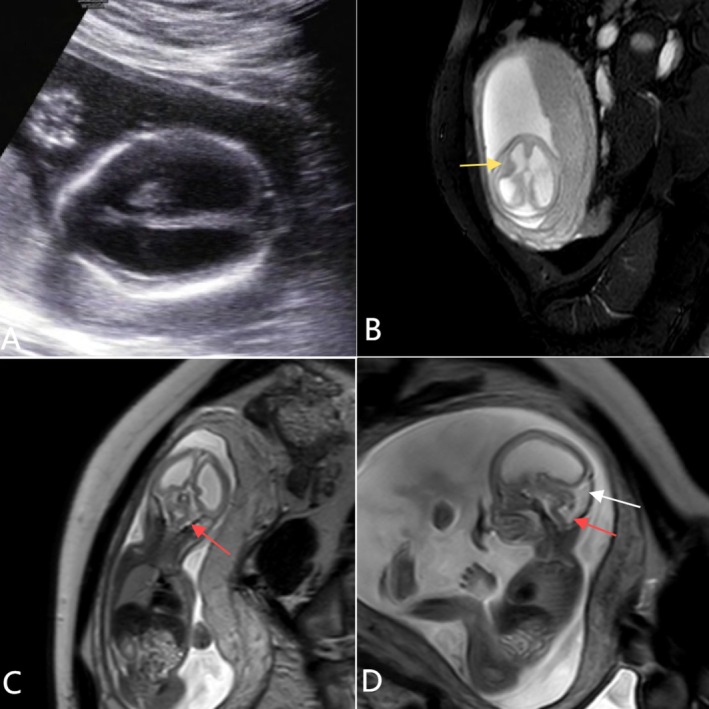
Images from Case 1. (A) US imaging at 19 weeks and 1 day shows severe triventricular hydrocephalus. Supratentorial sonographic images display an outer agyric echogenic band on the brain surface, along with a complete absence of the pericerebral space. (B–D) In vivo MRI at 22 weeks reveals axial T2—weighted imaging, which shows agyria and the absence of the pericerebral space, leading to interhemispheric pseudofusion. Severe ventriculomegaly is observed, along with periventricular cortical nodular heterotopias, indicating a neuronal migration disorder (yellow arrow). The sonographic midsagittal image shows a ‘Z’—shaped appearance of the brainstem (red arrows), agenesis of the corpus callosum, and a small vermis (white arrows).
